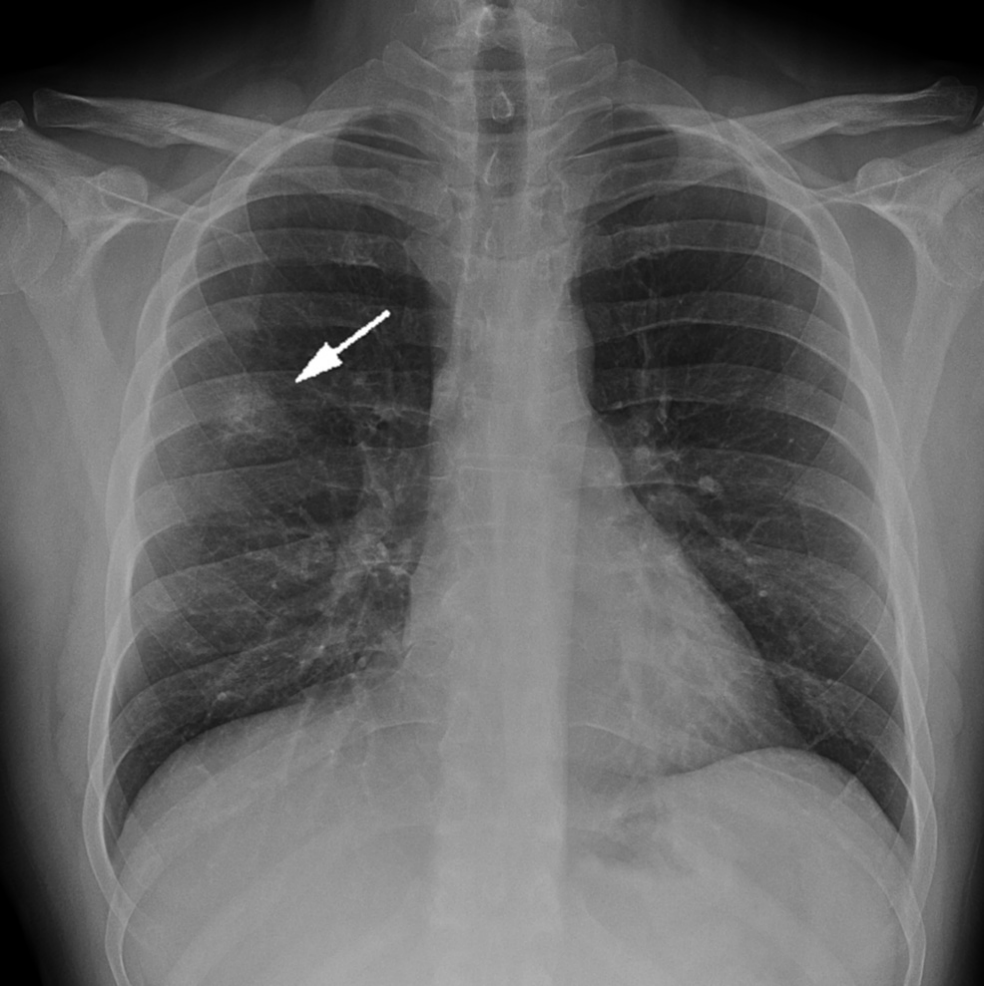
Chest X-ray A plain chest X-ray showing a solitary pulmonary nodule 2 cm in diameter (arrow) in the right mid-lung
